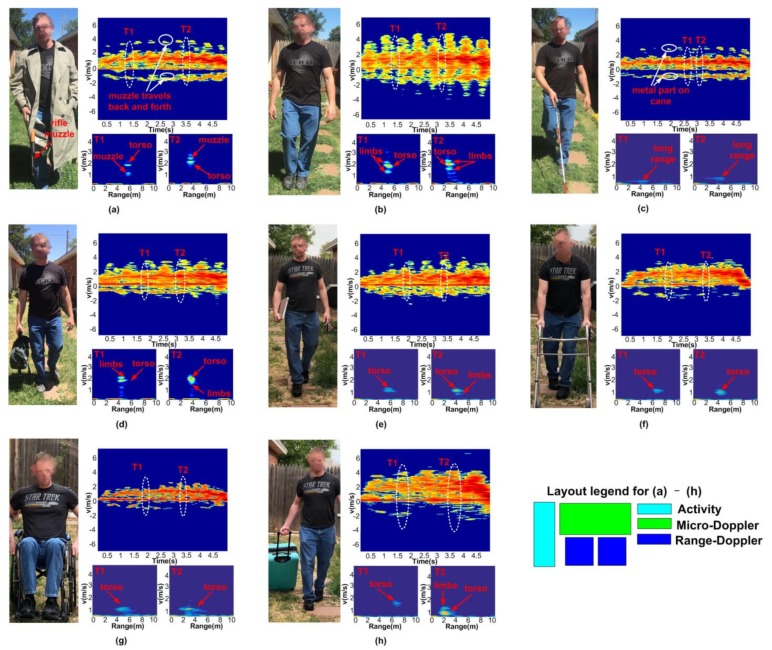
Micro-Doppler and range-Doppler properties of a human subject walking or moving at different conditions. (a) with a concealed rifle; (b) with natural arm swings; (c) with a cane for a blind person; (d) with holding a gym bag; (e) with carrying a laptop; (f) with a walker; (g) moving in a wheelchair; (h) with a rolling suitcase [27].
